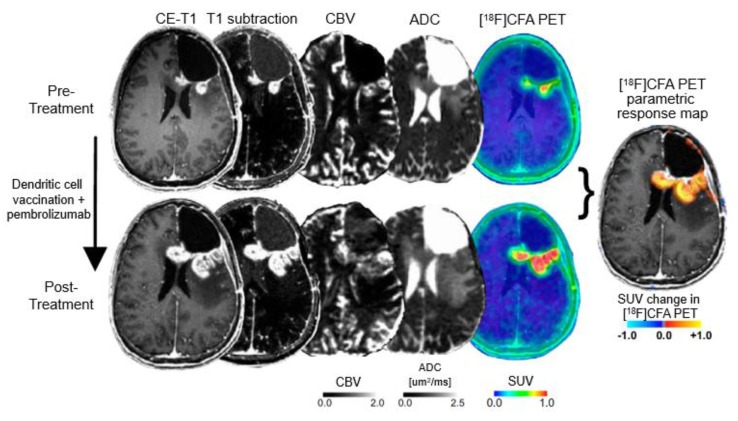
Detection of immune response in a patient with recurrent glioblastoma using 2-chloro-2′-deoxy-2′-[^18^F]fluoro-9-b-D-arabinofuranosyl-adenine ([^18^F]CFA) positron-emission tomography (PET) and advanced magnetic resonance imaging (MRI) before (upper panel) and after treatment with dendritic cell vaccination and programmed cell death receptor-1 (PD-1) blockade using pembrolizumab (lower panel). Following treatment, [^18^F]CFA uptake is considerably increased, indicating an immune-cell infiltration, and helps distinguishing tumor progression from inflammation (modified from Antonios et al. [169], with permission from the National Academy of Sciences).
